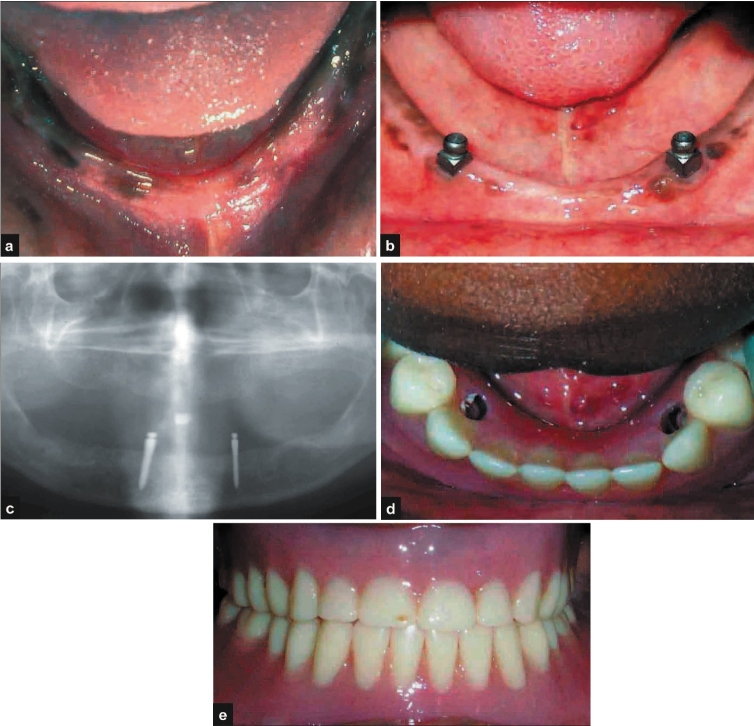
a) Pre-operative photograph; b-e) post-operative photographs and radiograph
